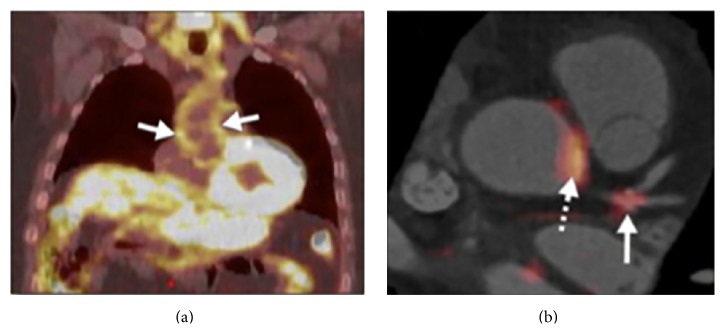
Imaging arterial inflammation using (a) FDG-PET patient demonstrating enhanced aortic uptake of FDG on PET scan, indicating inflammation in the arterial wall due to atherosclerosis. (b) Coregistered FDG-PET/computed tomography images showing FDG uptake at the left main coronary artery trifurcation (solid arrow) in a patient with acute coronary syndrome. Aortic FDG uptake is indicated by the dashed arrow. In such patients, both aortic and coronary artery FDG uptake was increased compared with patients with stable coronary artery disease. Reprinted with permission from Rudd et al. [47].
